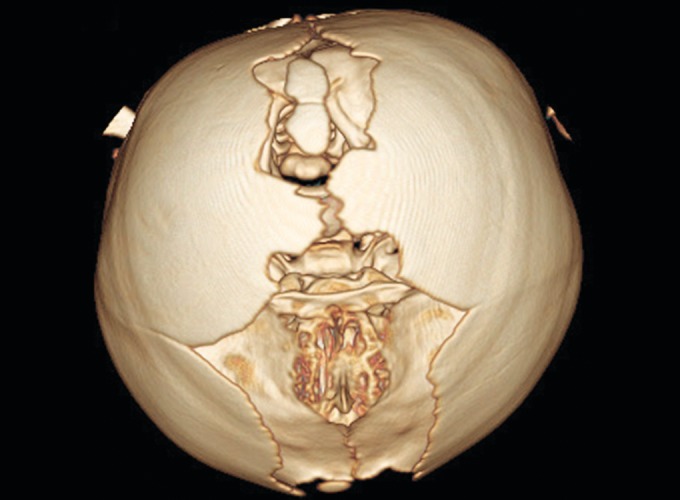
Three-dimensional CT showing a midline defect extending from glabella to posterior fontanelle with abnormally wide anterior and posterior fontanelle
